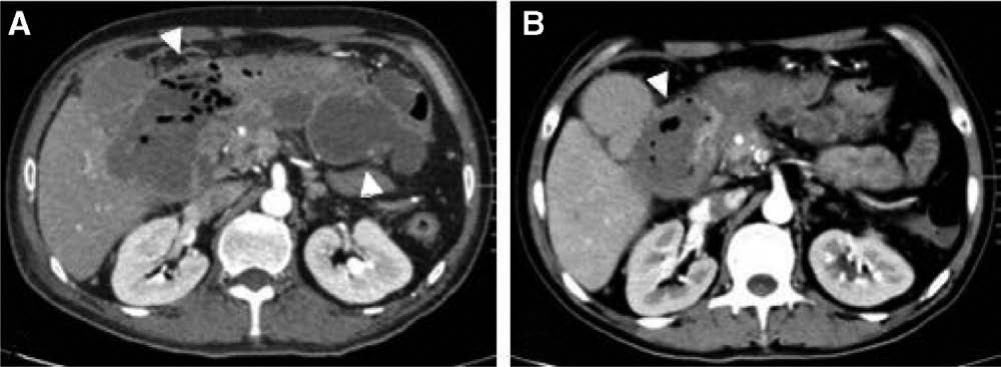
(A)Abdominal CT scan showed the presence of irregular encapsulated retroperitoneal infection containing much gas (white arrowhead). (B) CT scan 6 days after embolization showed significant regression in the size of the retroperitoneal infection (white arrowhead). CT = computed tomography.
